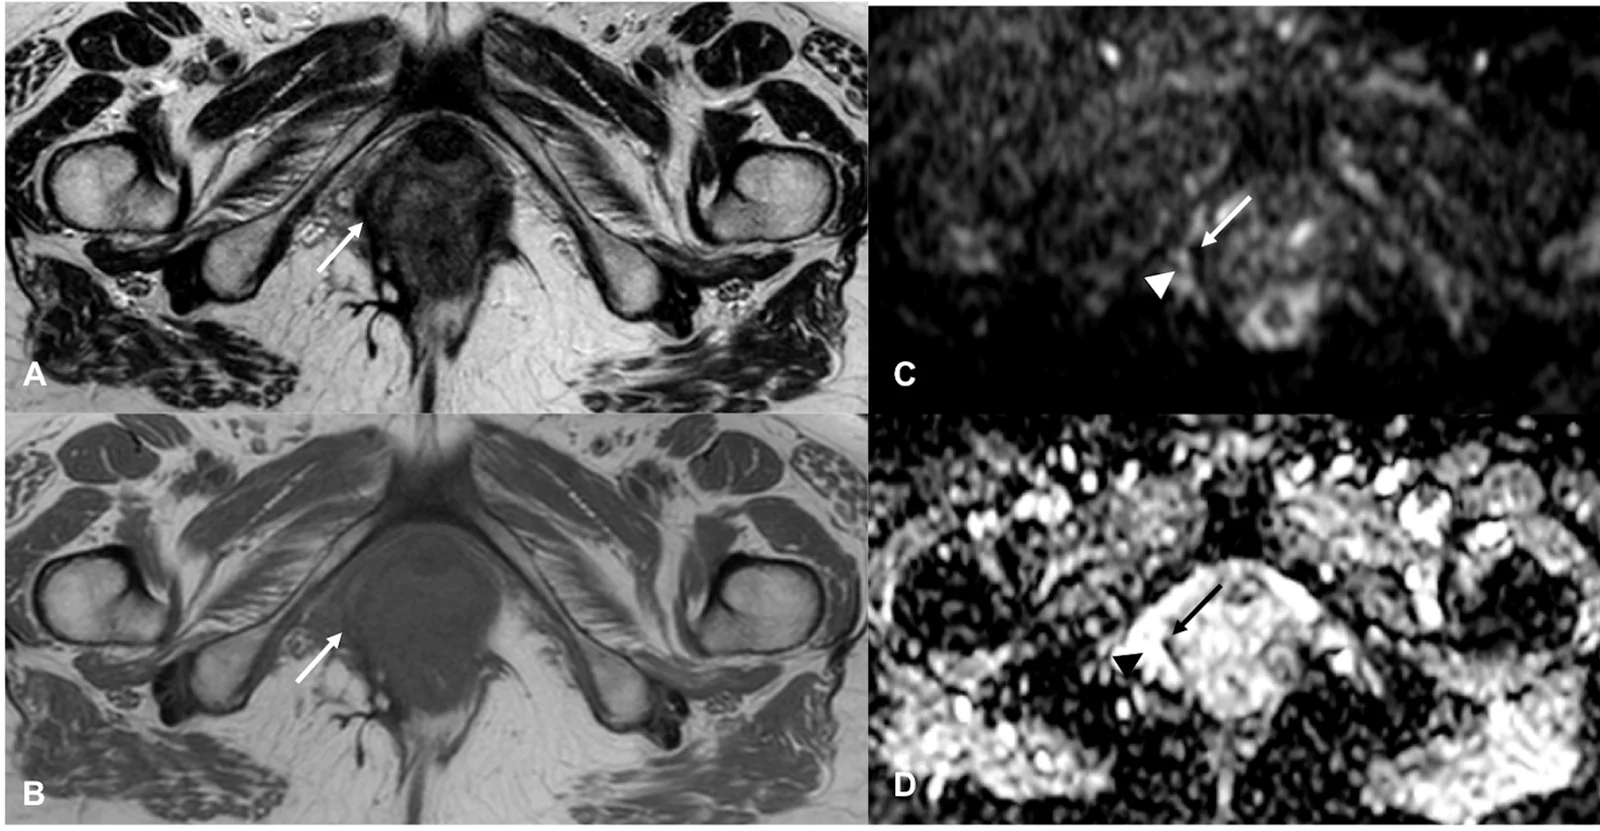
MRI appearance after eight years of continuous gonadotropin-releasing hormone (GnRH) agonist therapy A, B) Axial T2-weighted (A) and T1-weighted (B) images demonstrate complete radiologic tumor regression, with only postoperative fibrotic changes remaining (arrows). C, D) No diffusion restriction is demonstrated on diffusion-weighted imaging (DWI) (arrowhead in C), with corresponding high signal on the apparent diffusion coefficient (ADC) map (arrowhead in D), interspersed with low-signal-intensity fibrotic changes (arrows).
